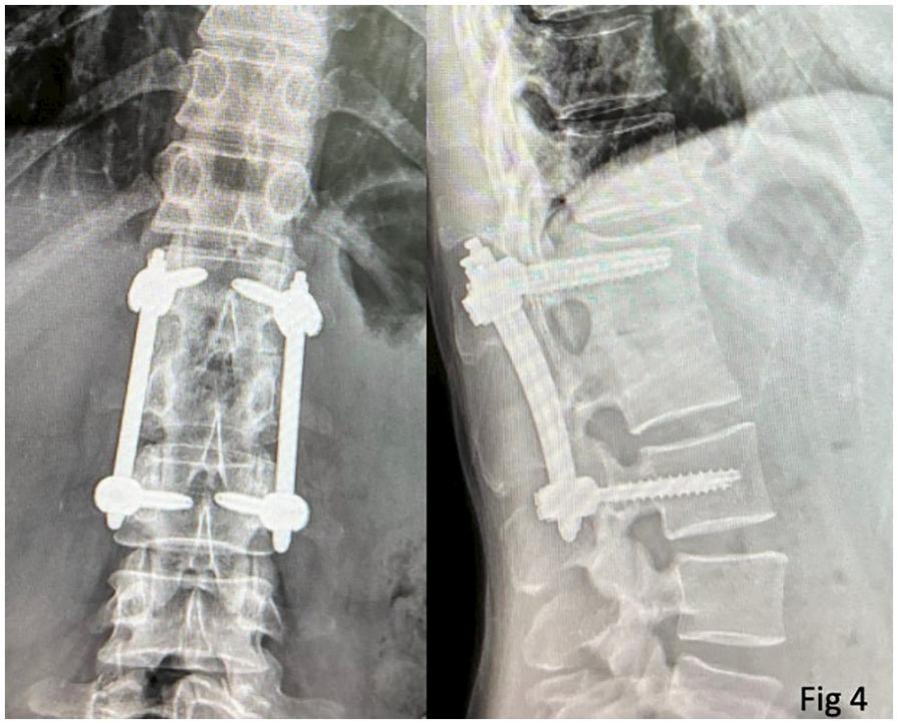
A radiograph of the patient’s thoracolumbar spine 2 years after the operation shows a fused L1/2 body and the absence of the previous cavity. The screws are well-fixed.
